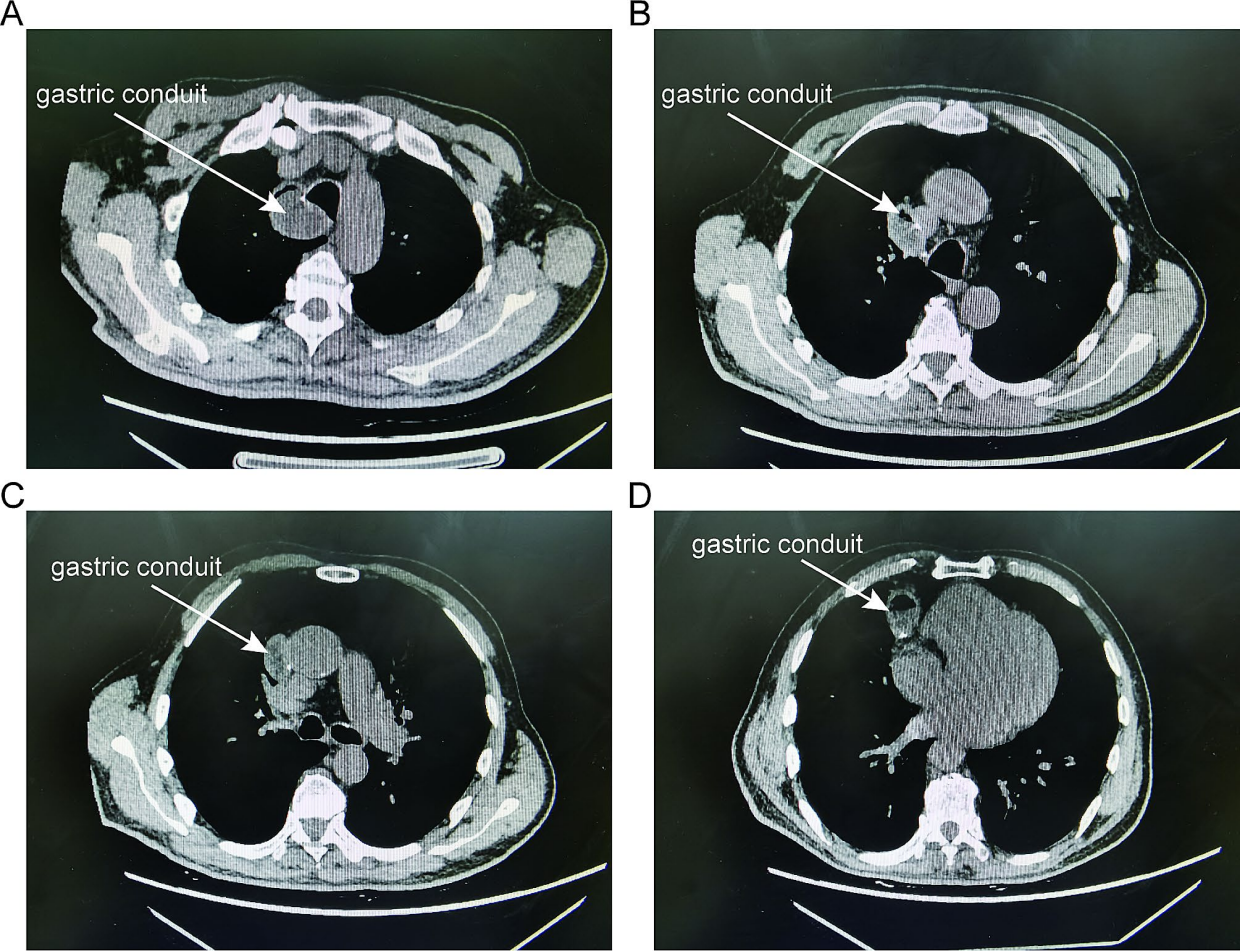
Radiographic images after surgery. (A-D) Axial images of postoperative chest computed tomography of the patient. The gastric conduit is passed across the anterior of the pulmonary hilum
